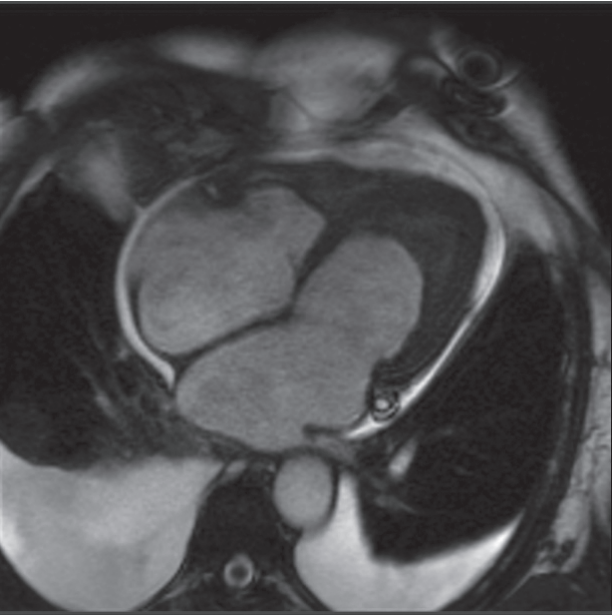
MRI Four Chamber View Through Both Ventricles Showed Apical Filling of Both Ventricles by Thrombus. Significant Pleural Effusion is Also Noted
